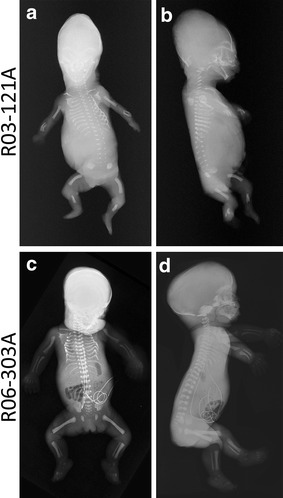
SRPS radiographic phenotype. a, b Radiographic findings in R03-121A. An 18-week gestational age fetus with short, narrow bent ribs, a bell-shaped chest, handlebar clavicles, curved humeri with short bent radii and ulnae, curved femurs, fibular aplasia and lack of calcification of the distal extremities. c, d Radiographic findings in R06-303A. 30-week gestational age fetus with dolichocephaly and a prominent occiput, a long narrow thorax with deformed ribs, micromelia, and poor mineralization of the distal limbs, mild platyspondyly, and abnormal ilia
